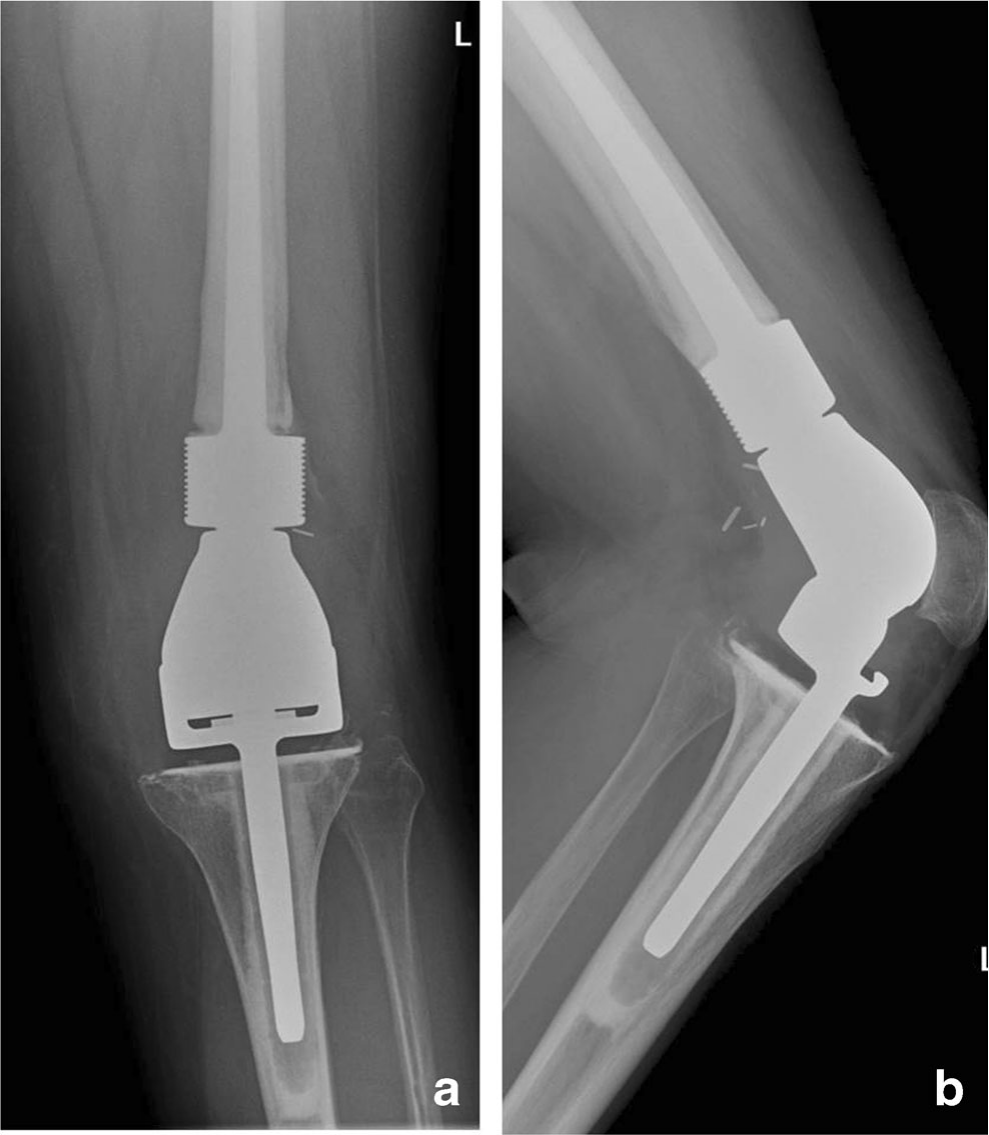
a Anteroposterior and b lateral plain radiographs post-resection of the distal femur and proximal tibia, with insertion of a distal femoral endoprosthesis, coupled to a rotating hinged tibial component
